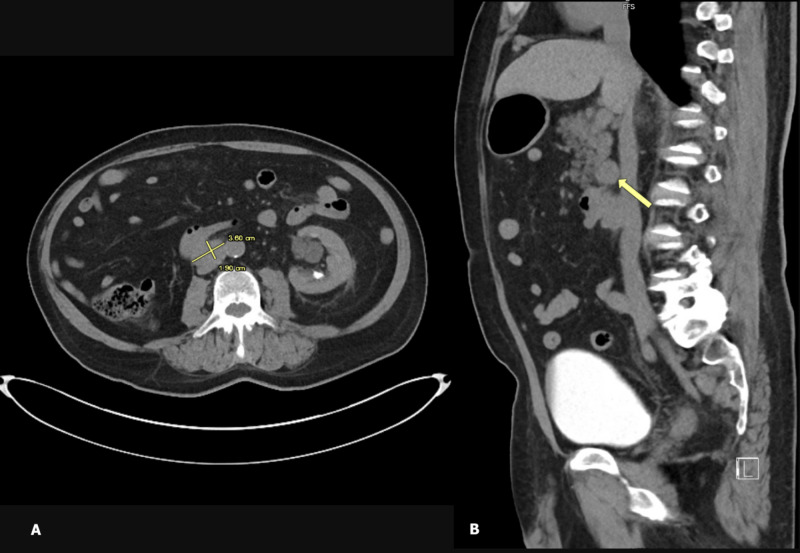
CTAP showing an enlarged LN anterior to the inferior vena cava – measuring 3.6 cm x 1.9 cm in largest dimension on (A) transverse section (dimensions marked in yellow) and (B) sagittal section (arrow). CTAP, computed tomography abdomen and pelvis; LN, lymph node
